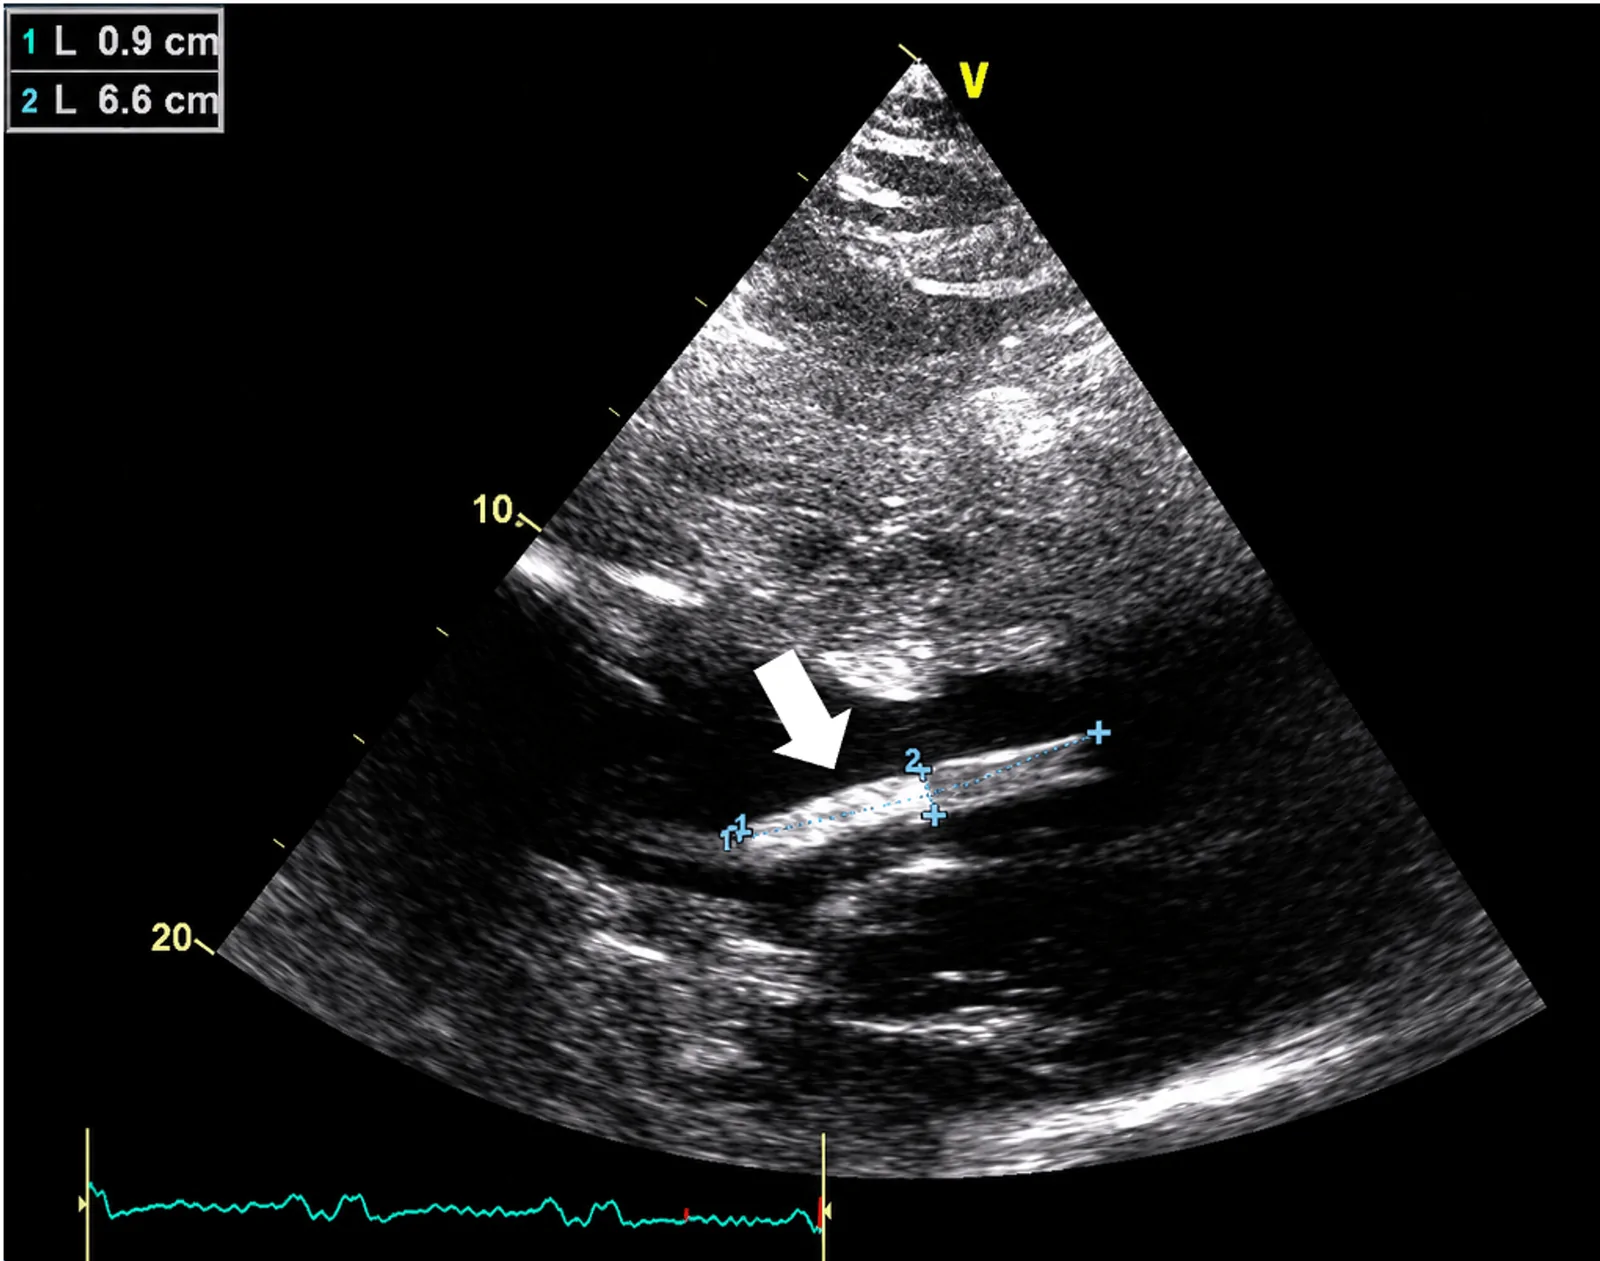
Transthoracic echocardiography showing a linear echogenic foreign body within the right-sided cardiac chambers, consistent with a migrated venous stent measuring approximately 66 × 9 mm. The arrow indicates the migrated stent.
